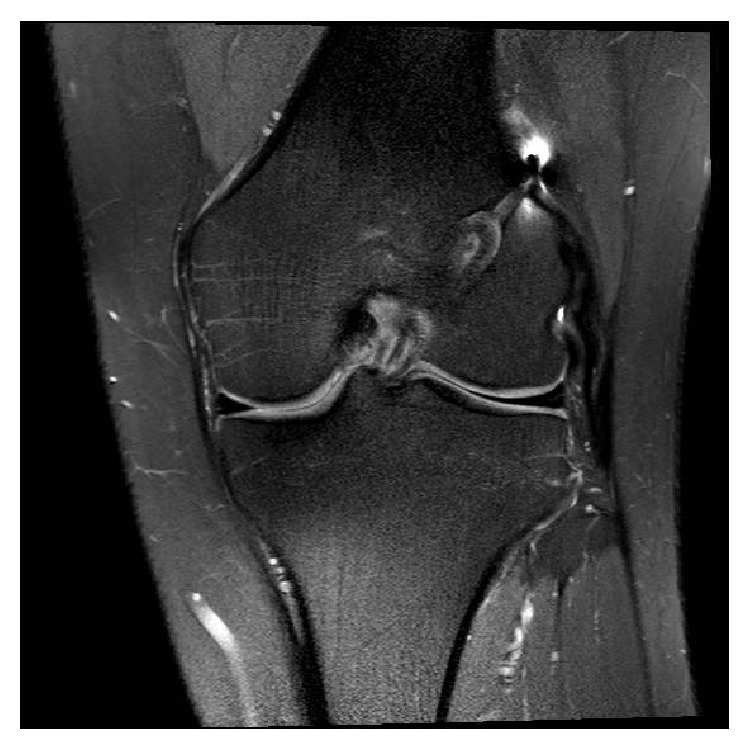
Postoperative coronal MRI scan of the left knee at 16 months demonstrating an intact, well-tensioned reconstruction with no signs of complications.
